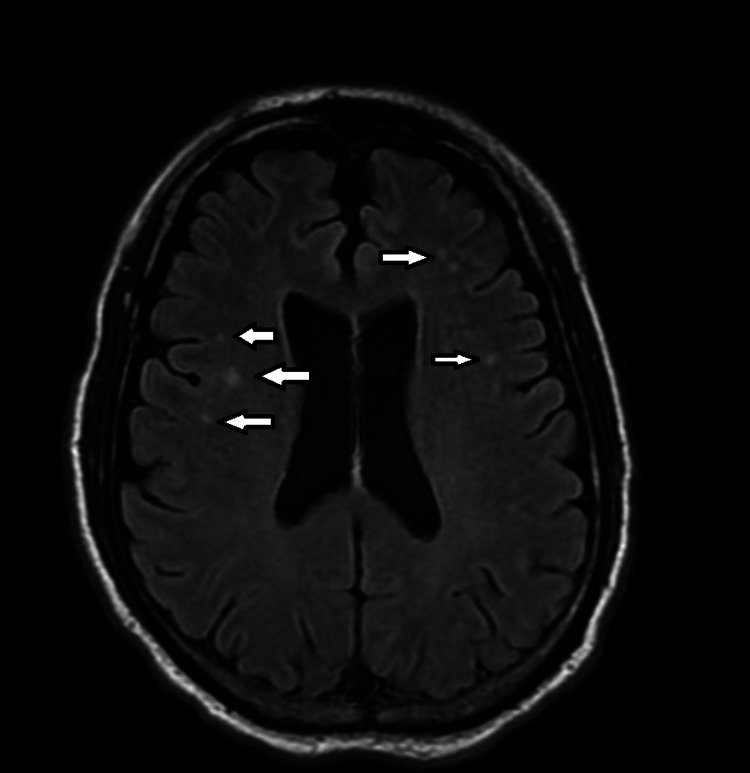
T2 FLAIR MRI without contrast showing punctate hyperintensities (white arrows) distributed in the deep cortical regions, 2024. This distribution is more consistent with changes seen in migraine than age-related changes, which are most often periventricular.
